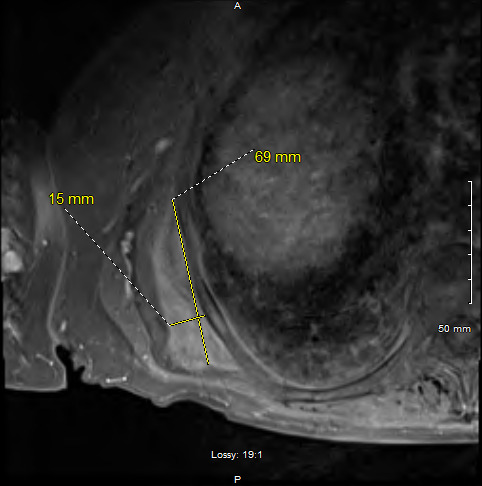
MRI of the Scapula. Mass deep to the right latissimus dorsi and serratus anterior muscles at and extending inferior to the distal tip of the scapula. This measures 6.9 x 1.5 cm, composed of soft tissue components of similar signal intensity in the skeletal muscle with small foci of intermixed fat and mild heterogeneous postcontrast enhancement. Impression of the exam was consistent with elastofibroma dorsi. [A:Anterior, S: Superior].
